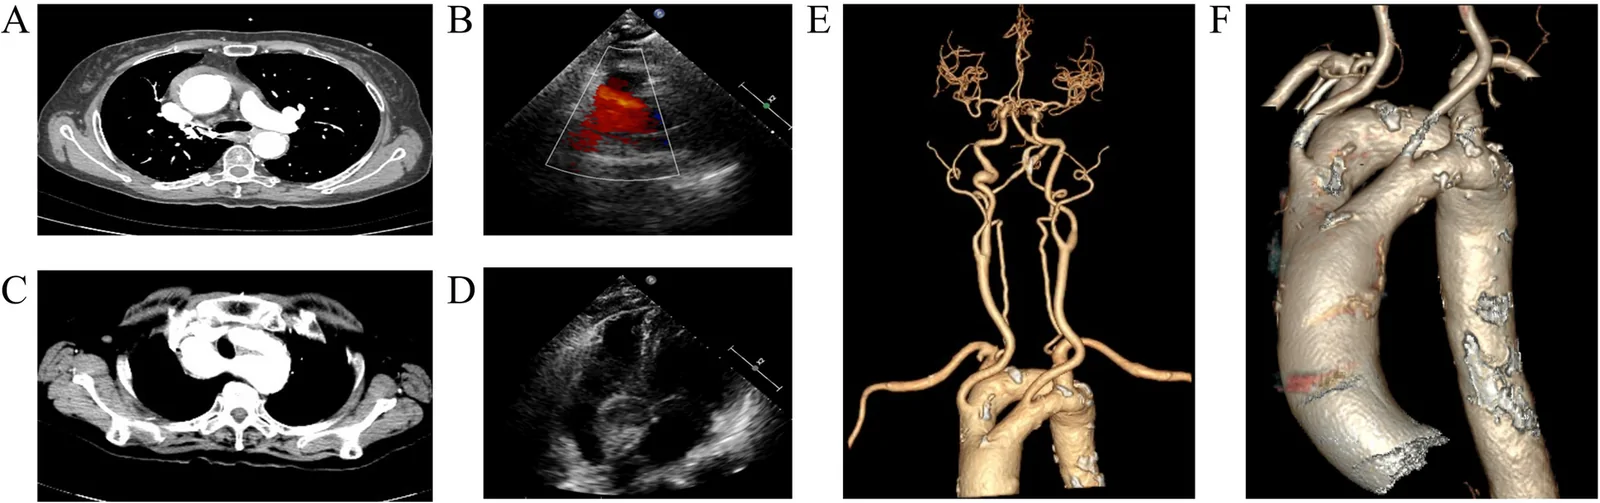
A, C Emergency CTA demonstrates a double aortic arch; an ulcer-like lesion in the ascending aorta suggests possible PAU. B, D Echocardiography shows no concomitant congenital cardiac malformation. E, F Reconstructed images highlight the complex supra-aortic branch anatomy
